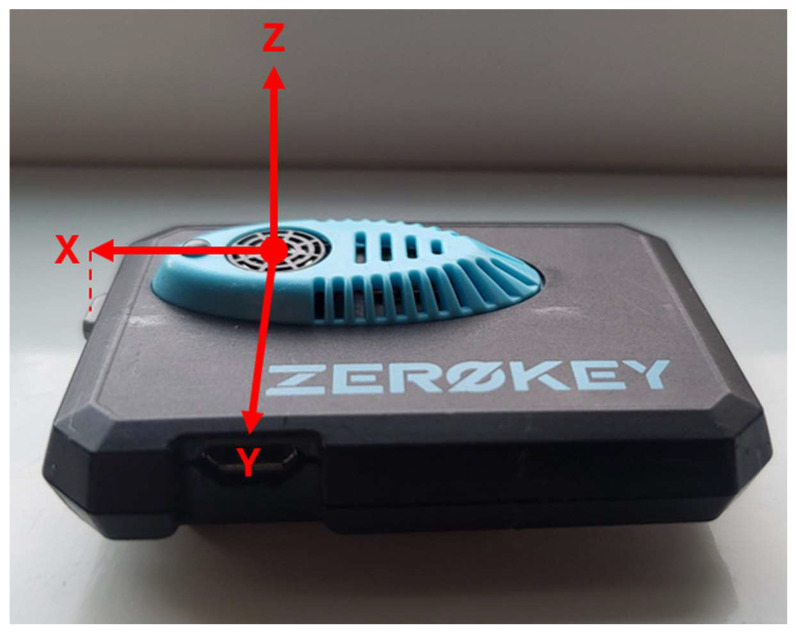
ZeroKey mobile node body frame coordinate system. The origin is located at the center of the ultrasonic transducer, with the positive X-axis pointing toward the action button, the positive Y-axis pointing toward the micro-USB port, and the Z-axis completing a right-handed coordinate system. Adapted from [28].
